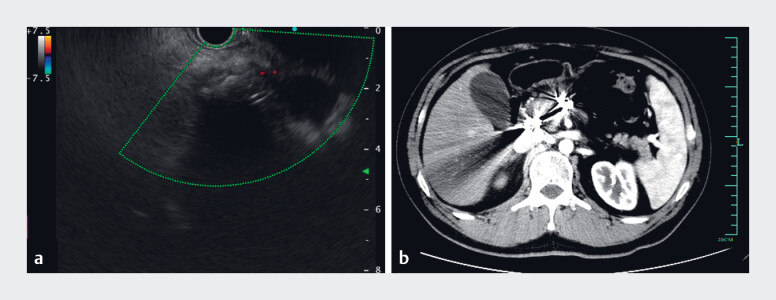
EUS and CT images at the 6-month follow-up after EUS-guided embolization.
